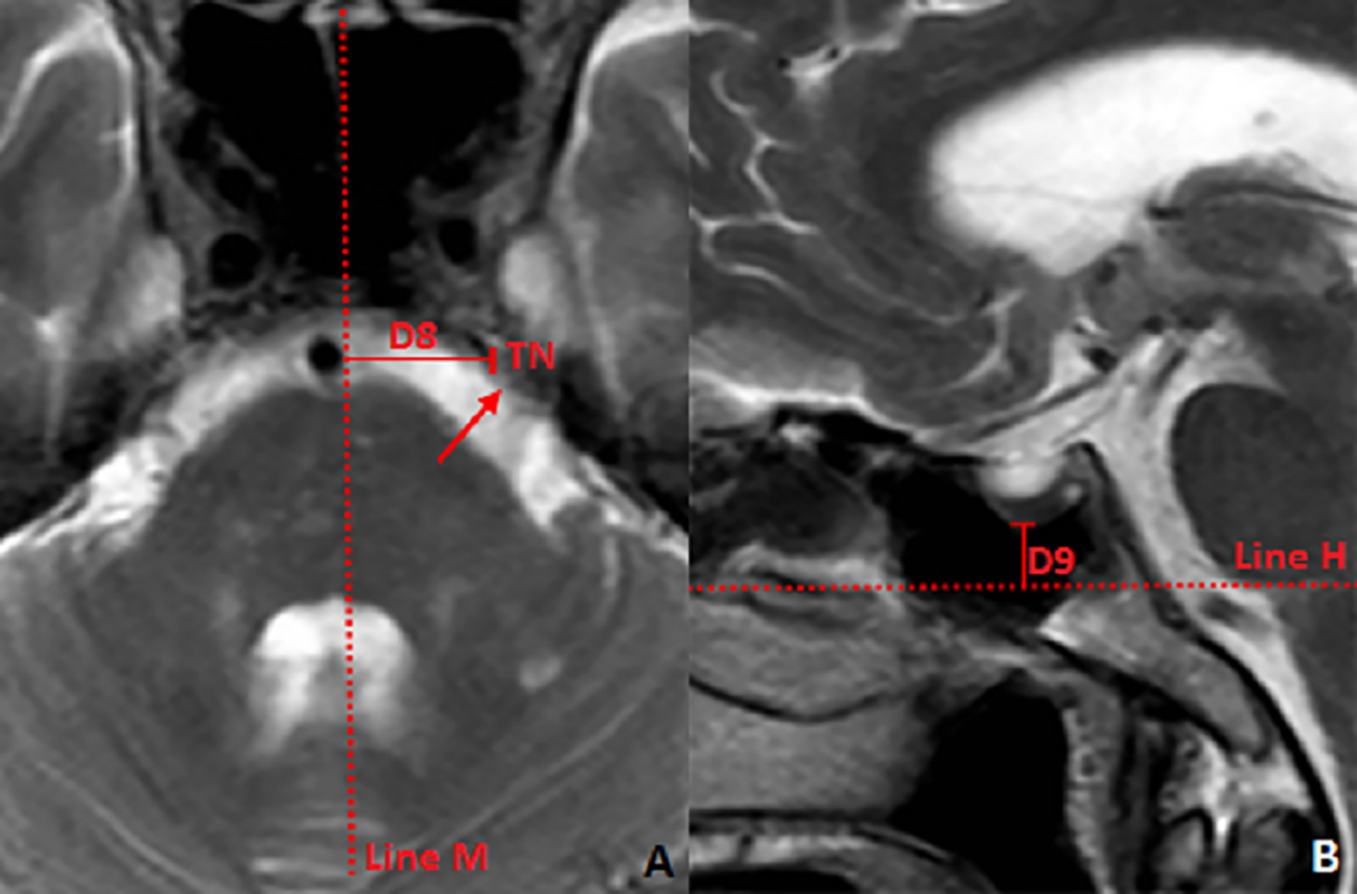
Location of trigeminal nerve in the MR image. 9A: Distance from the initial part of the trigeminal nerve to the mid-sagittal line; Line M: mid-sagittal line; TN: trigeminal nerve; D8: distance between the root of the trigeminal nerve and the mid-sagittal line. 9B: Line S: Position of the axial plane in plane 8A; PG: the pituitary gland; D9: the distance between the axial plane through the root of trigeminal nerve and lowest, central point of the SF.
